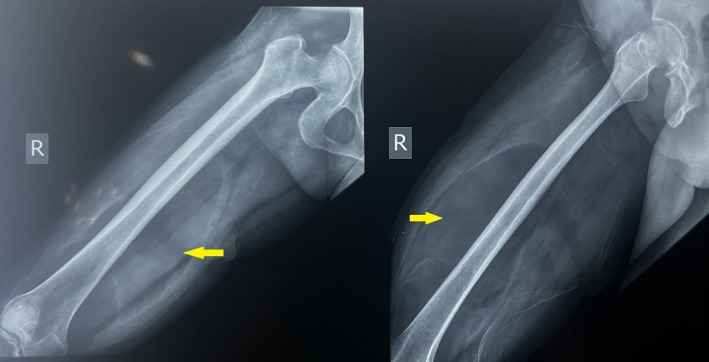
Plain radiographs of the right thigh in anteroposterior and lateral views demonstrate a well‐defined soft tissue radiolucent area along the medial to anteromedial aspect of the thigh (as indicated by yellow arrows). The lesion appears as an oval, homogeneous region of decreased soft tissue density consistent with fat attenuation.
